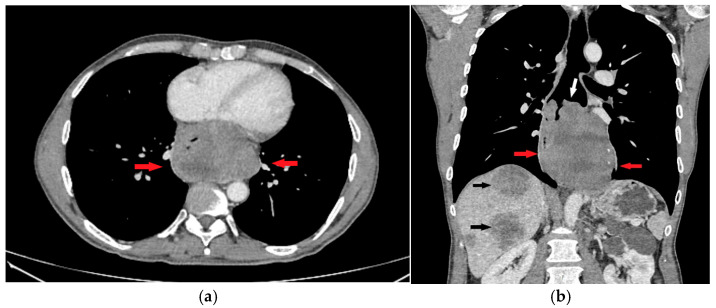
Axial (a) CT scan demonstrated an oval, predominantly solid mass with zones of initial necrotic degradation (red arrows), obliterating the esophageal lumen (b, white arrow). In liver parenchyma, multiple solid metastatic lesions can be observed (b, black arrows). Several enlarged, conglomerated lymph nodes were noted in the celiac, paraaortic, and hepatoduodenal regions.
